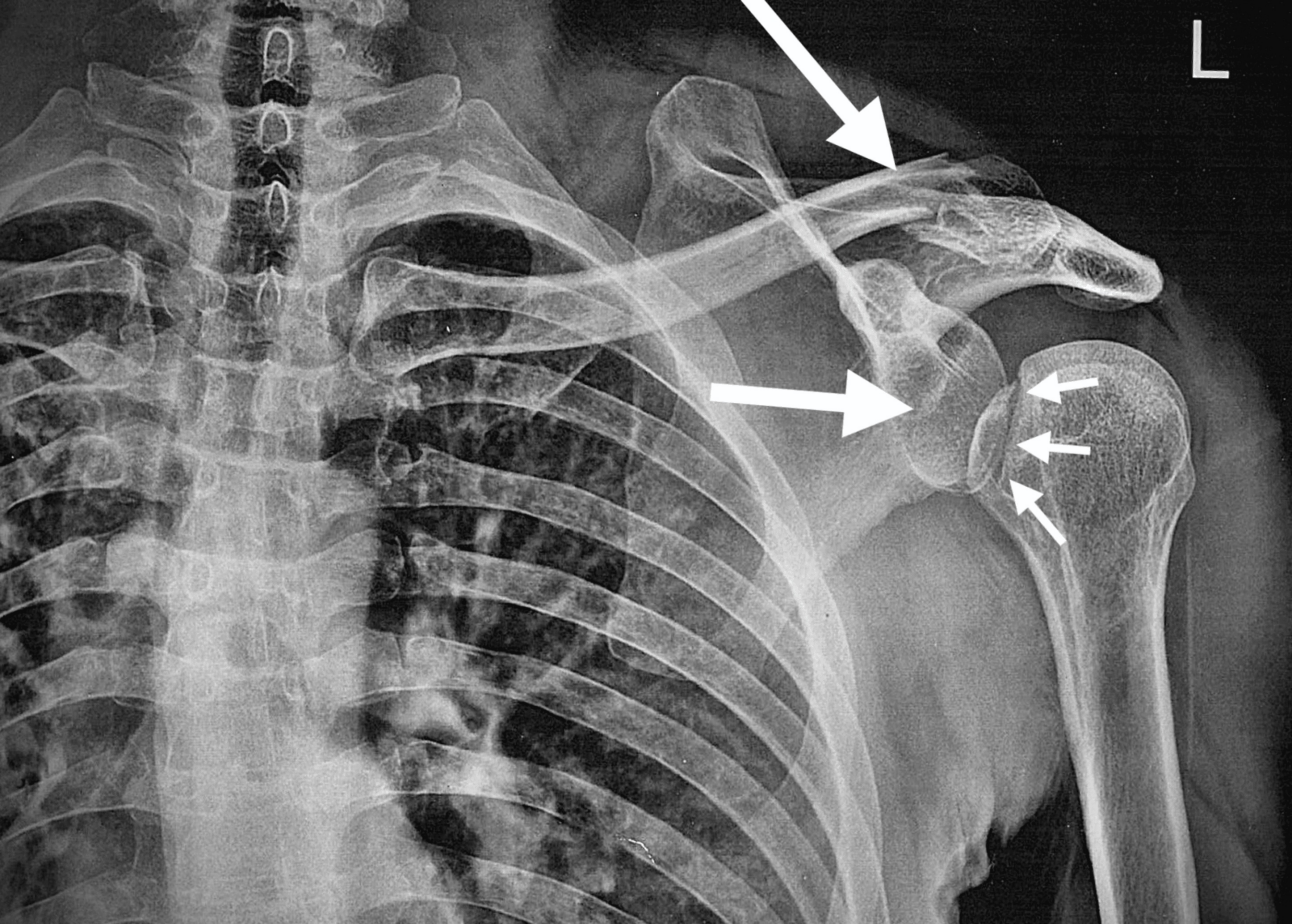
Anteroposterior X-ray of the left shoulder showing the posterior dislocation, fracture in the outer part of the clavicle, and a reverse Hill-Sachs lesion.
